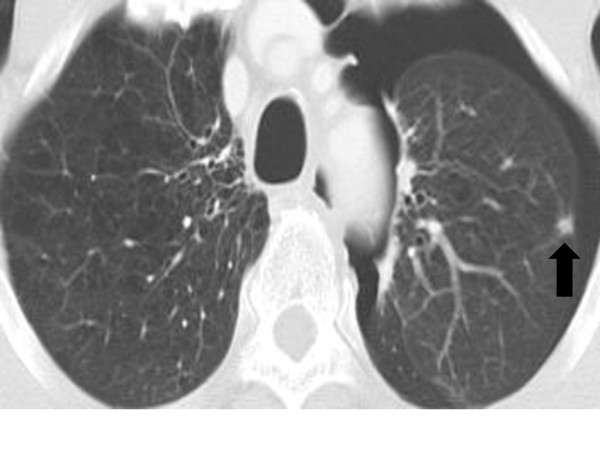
A follow-up CT scan showing a left pneumothorax, which developed secondary to pulmonary metastasis (arrow).
